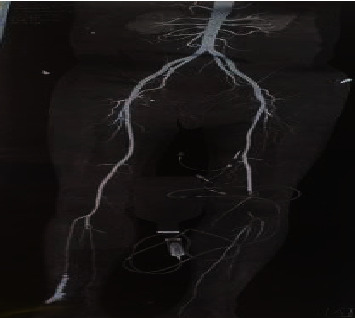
CT angiogram of abdominal aorta and bilateral lower limbs showing partially occluded thrombus in bilateral profunda femoris artery, completely occluding thrombus in left popliteal artery and tibioperoneal trunk.
